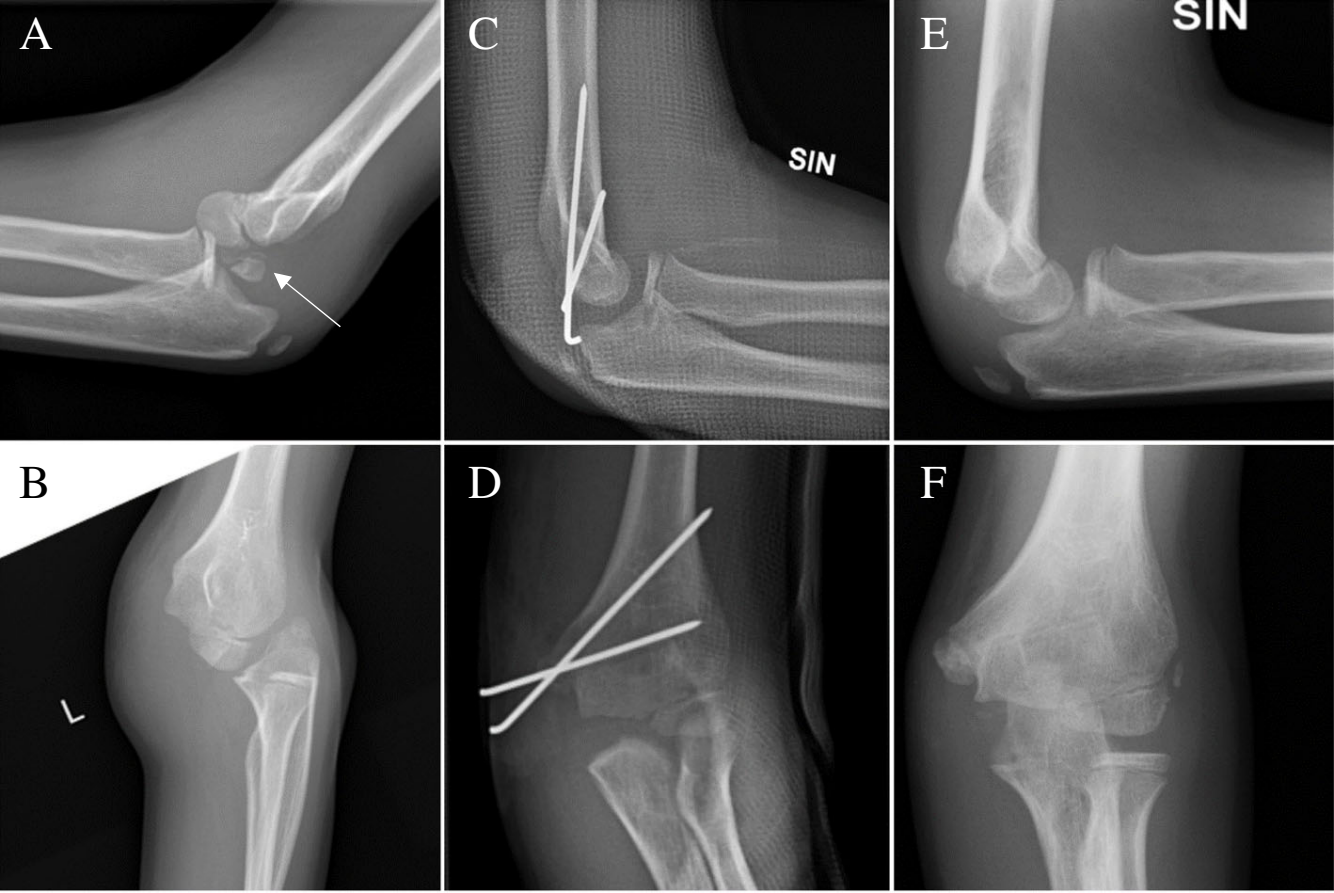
A Boy, 10.8 years old, was injured during trampoline jumping. Lateral (a) and anterior-posterior/oblique (b) radiographs of the left elbow showed a posterior-lateral dislocation and an avulsion of medial epicondyle (the arrow). Closed reduction was carried out in an emergency unit immediately and the fracture was fixed with K-wires (c−d). The elbow was immobilized with an above-the-elbow cast for 31 days. In the last follow-up visit the medial epicondyle was in good reduction. Functional and cosmetic results of the left elbow were satisfactory (e−f)
